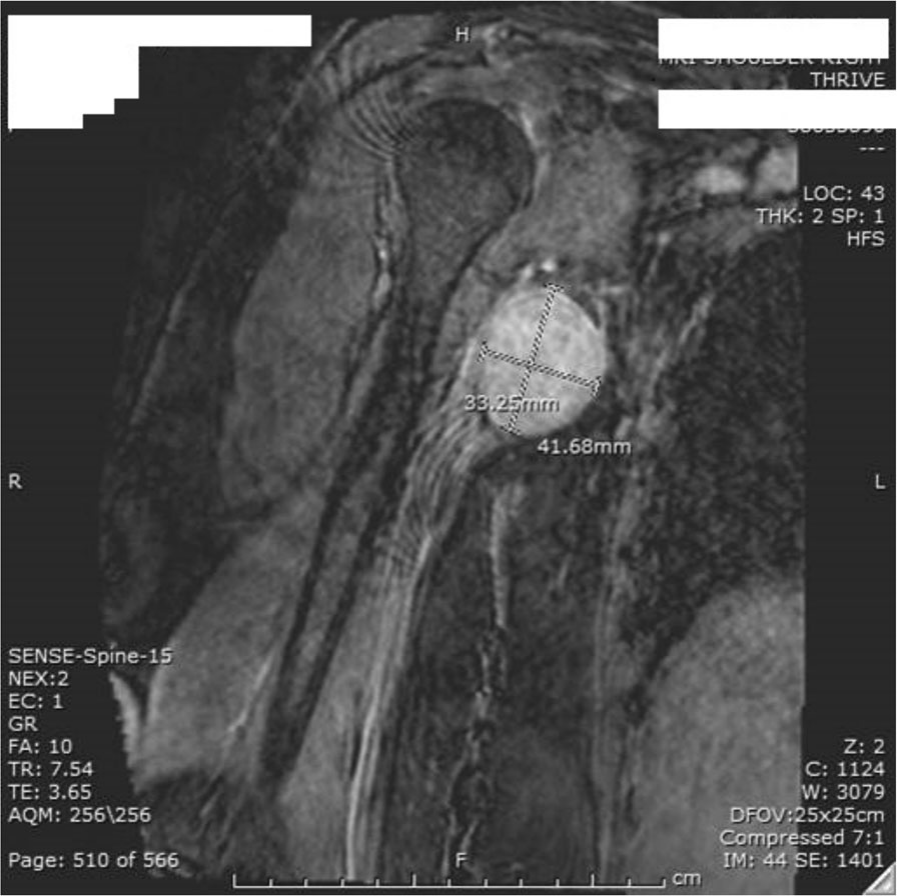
MRI (coronal view of the heterogeneous axillary mass)
